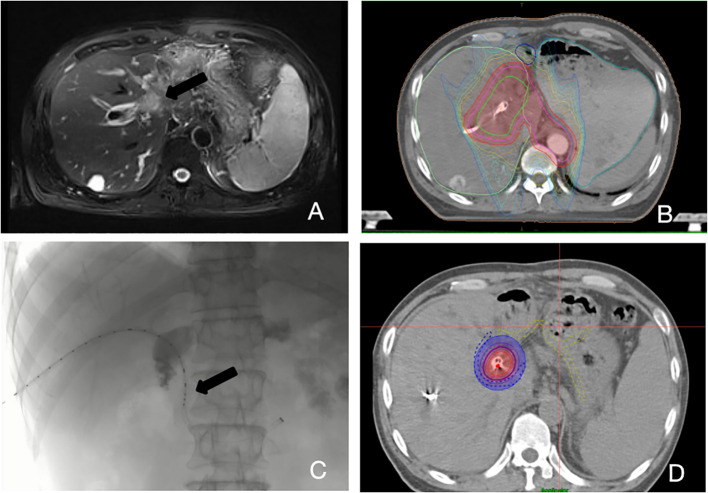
Representative images of the treatment procedure. (A) Contrast-enhanced CT image of type IV hilar cholangiocarcinoma, with the arrow indicating the tumor lesion; (B) fluoroscopic image after percutaneous biliary stent implantation; (C) fluoroscopic image of preplaced afterloading catheter with dummy source positioning, with the arrow indicating the biliary stent; (D) axial dose distribution map of ^192^Ir brachytherapy.
